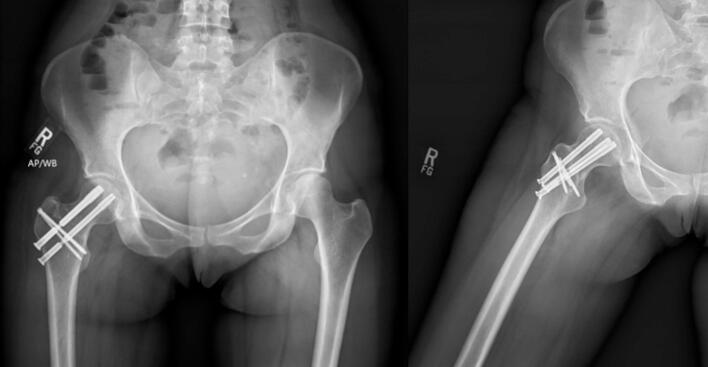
Postoperative AP and lateral radiographs from the patient's 10-week follow-up appointment demonstrating persistent anatomic reduction with no evidence of motion or radiolucency about the partially threaded screws across the fracture nor the upper screws that were inserted in the slotted holes.
